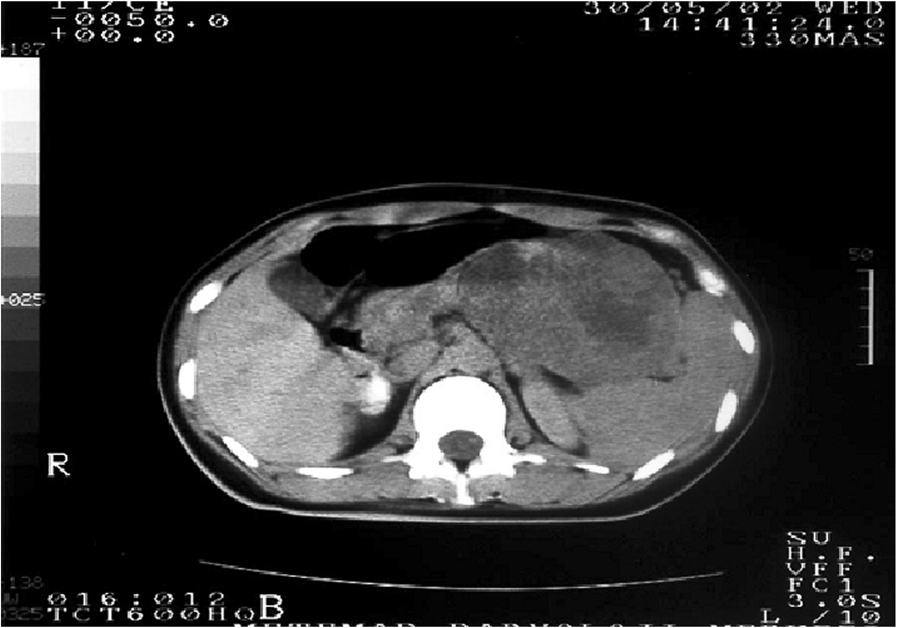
Magnetic resonance imaging shows that the tumor is a well-marginated, large, encapsulated, solid and cystic mass with areas of hemorrhagic degeneration, as revealed by high signal intensity.
